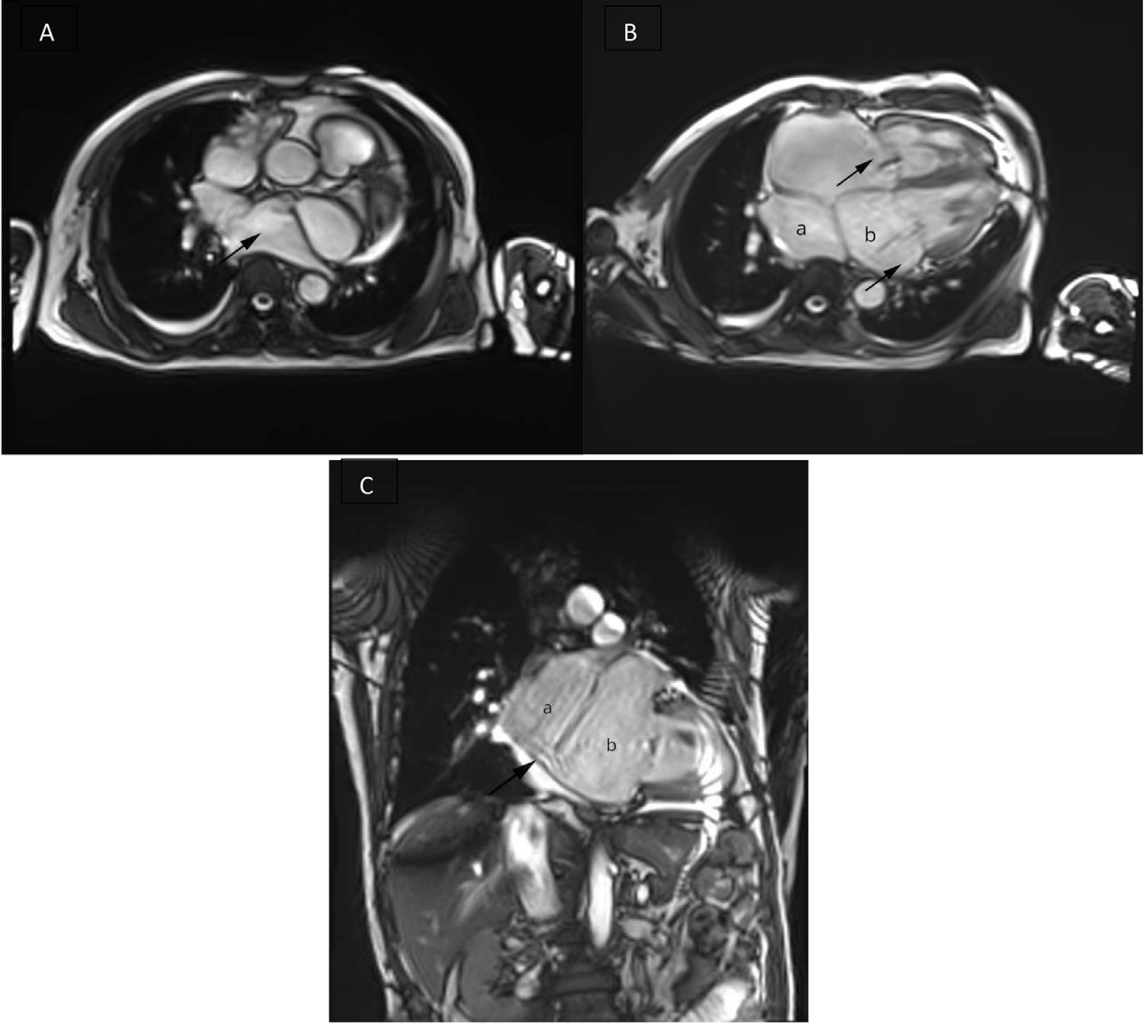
Cardiac MRI. (A) The left atrium branches into the pulmonary artery (arrow). (B) The left atrium has two chambers. Mitral and tricuspid regurgitation was seen (arrow). (C) Slice of 2 chambers. There is a defect between left atrium and extra atrium + 1.10 (arrow).
